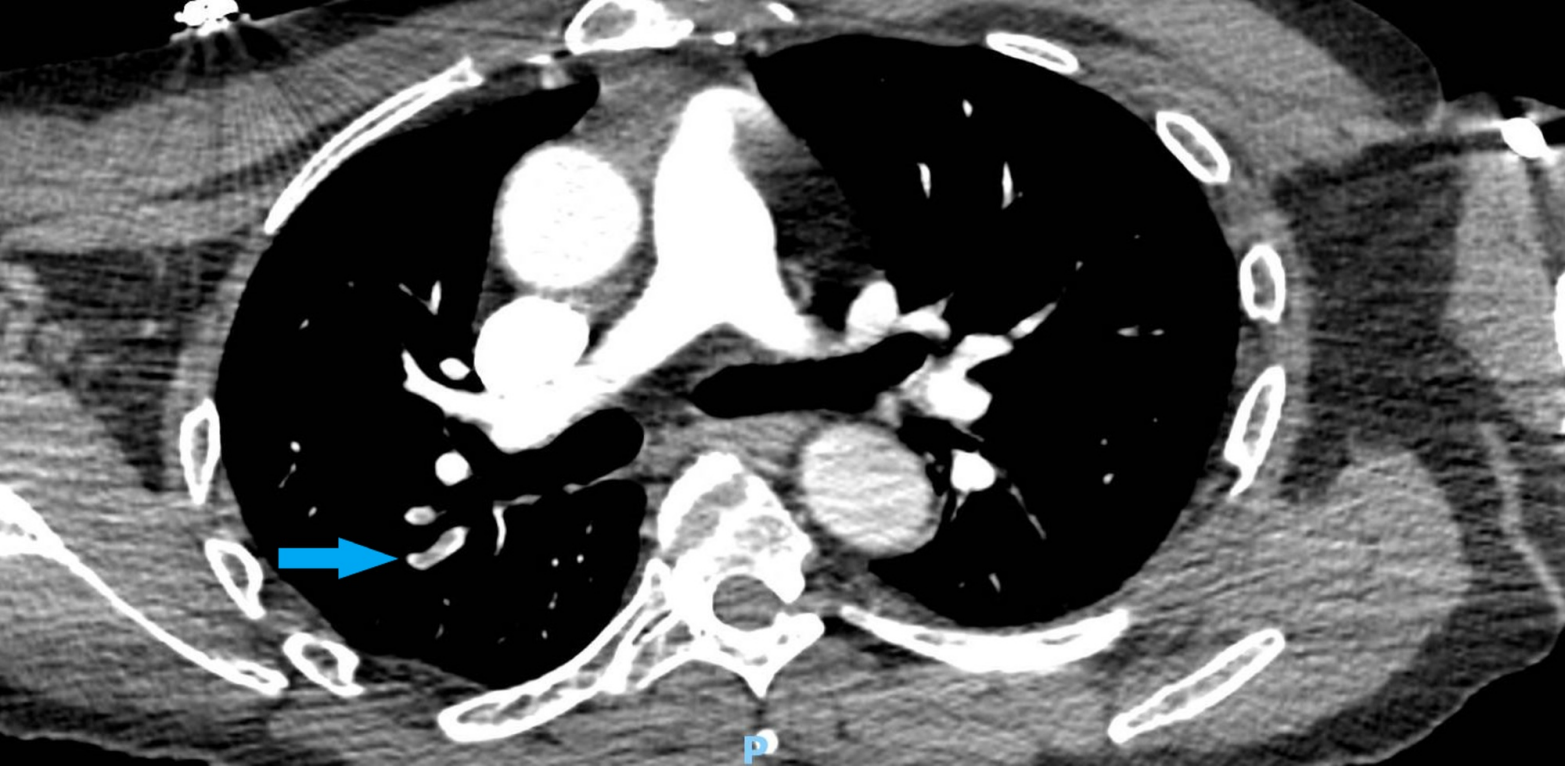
Computed tomography of the chest showing pulmonary embolism
